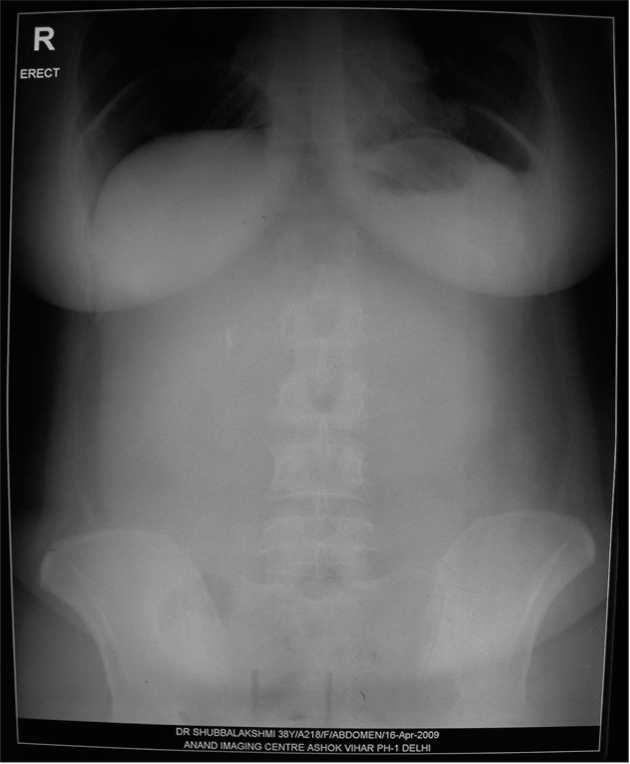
Radiograph of the abdomen (erect) showing free gas under diaphragm
